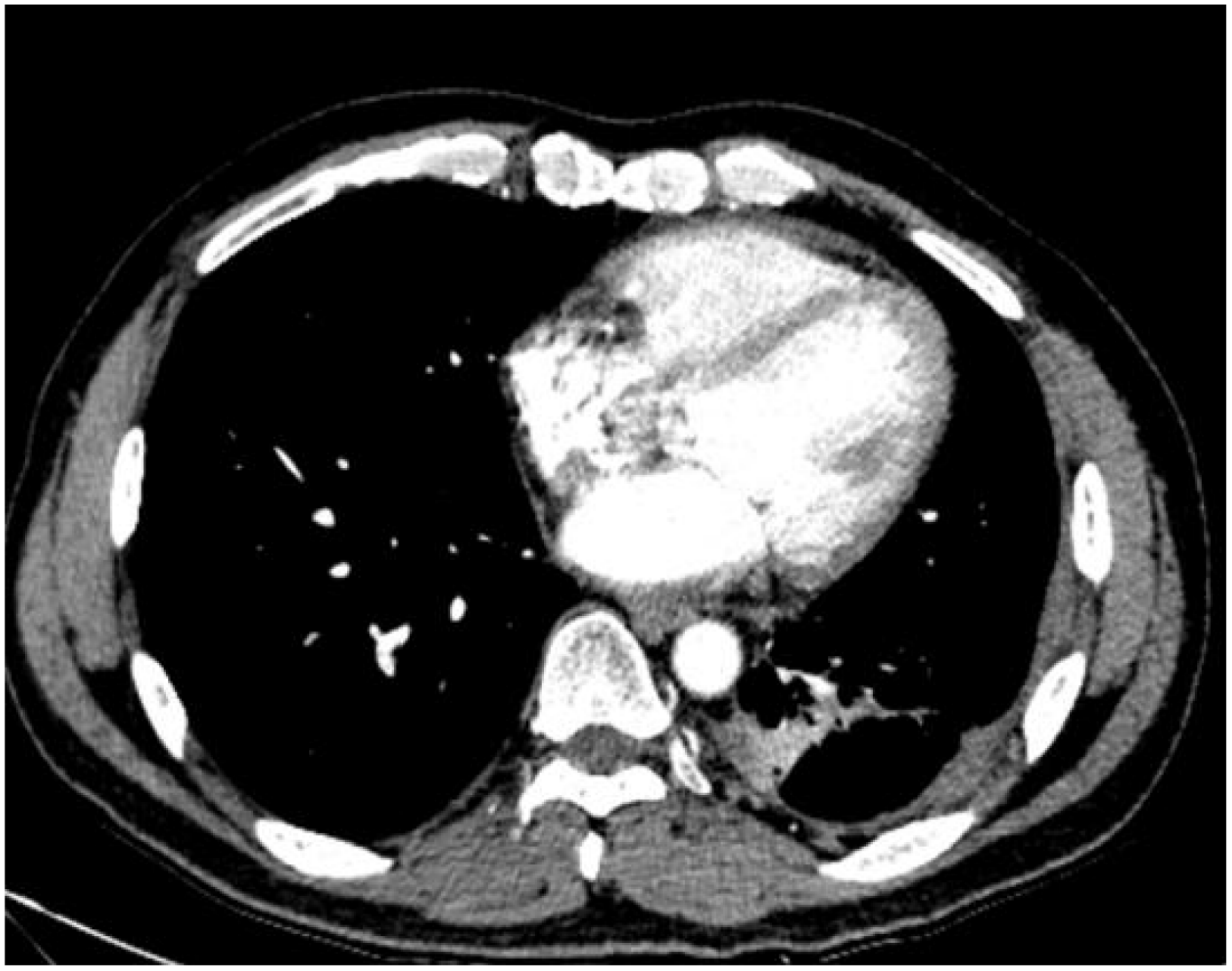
The chest CT scan performed on September 13, 2023, reveals central type lung cancer in the left lower lung with pulmonary metastases, accompanied by cancerous lymphangitis affecting both lungs and improved left lower lung collapse compared to earlier scans. There is also a reduction in metastases to the mediastinal and left pulmonary hilum lymph nodes. Additionally, left pleural metastasis with encapsulating pleural effusion shows improvement compared to previous imaging. A stable solid nodule is noted in the inner basal segment of the right lower lobe, and pericardial effusion has slightly decreased compared to prior examinations.
